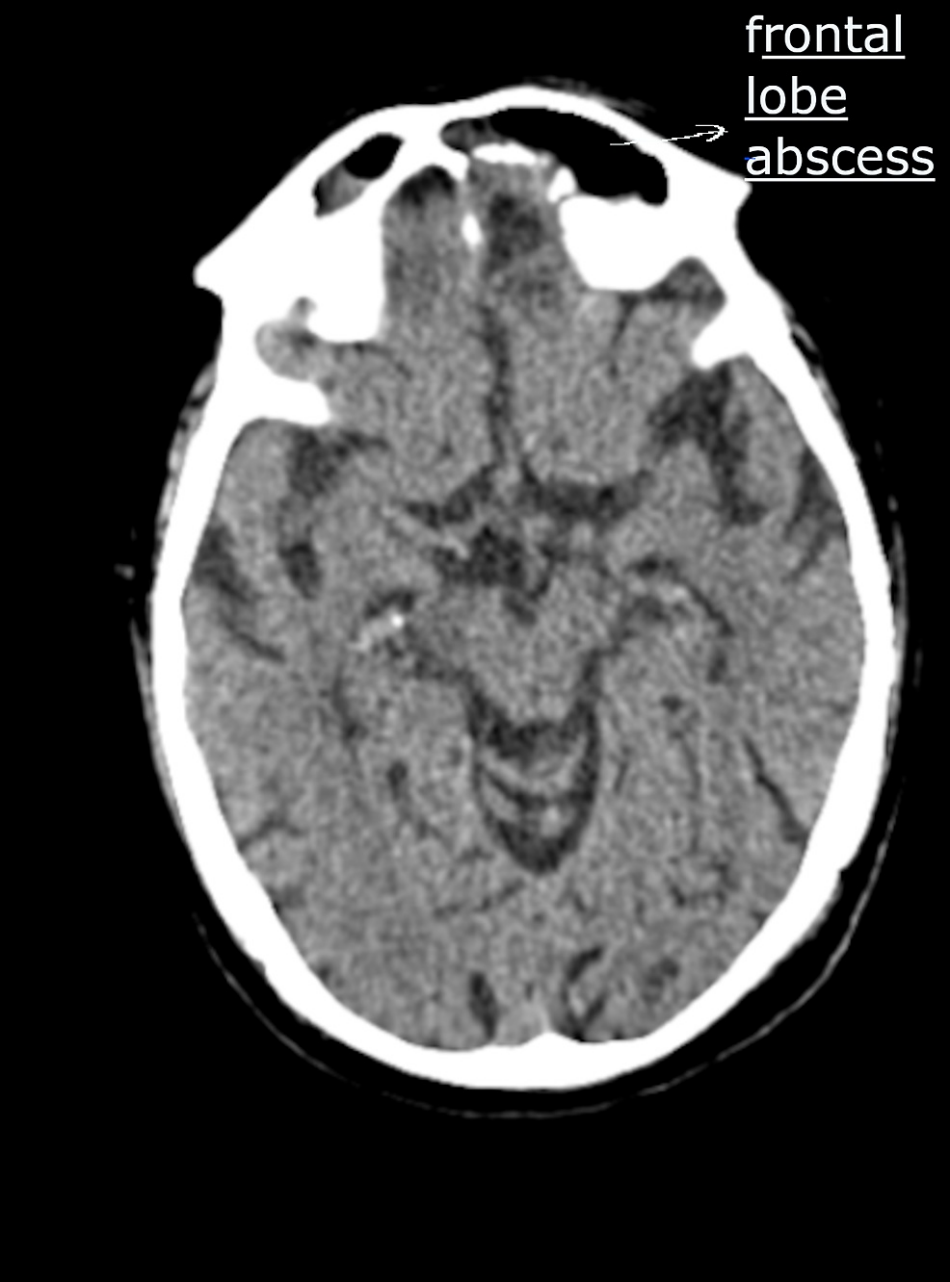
CT brain showing frontal lobe abscess
